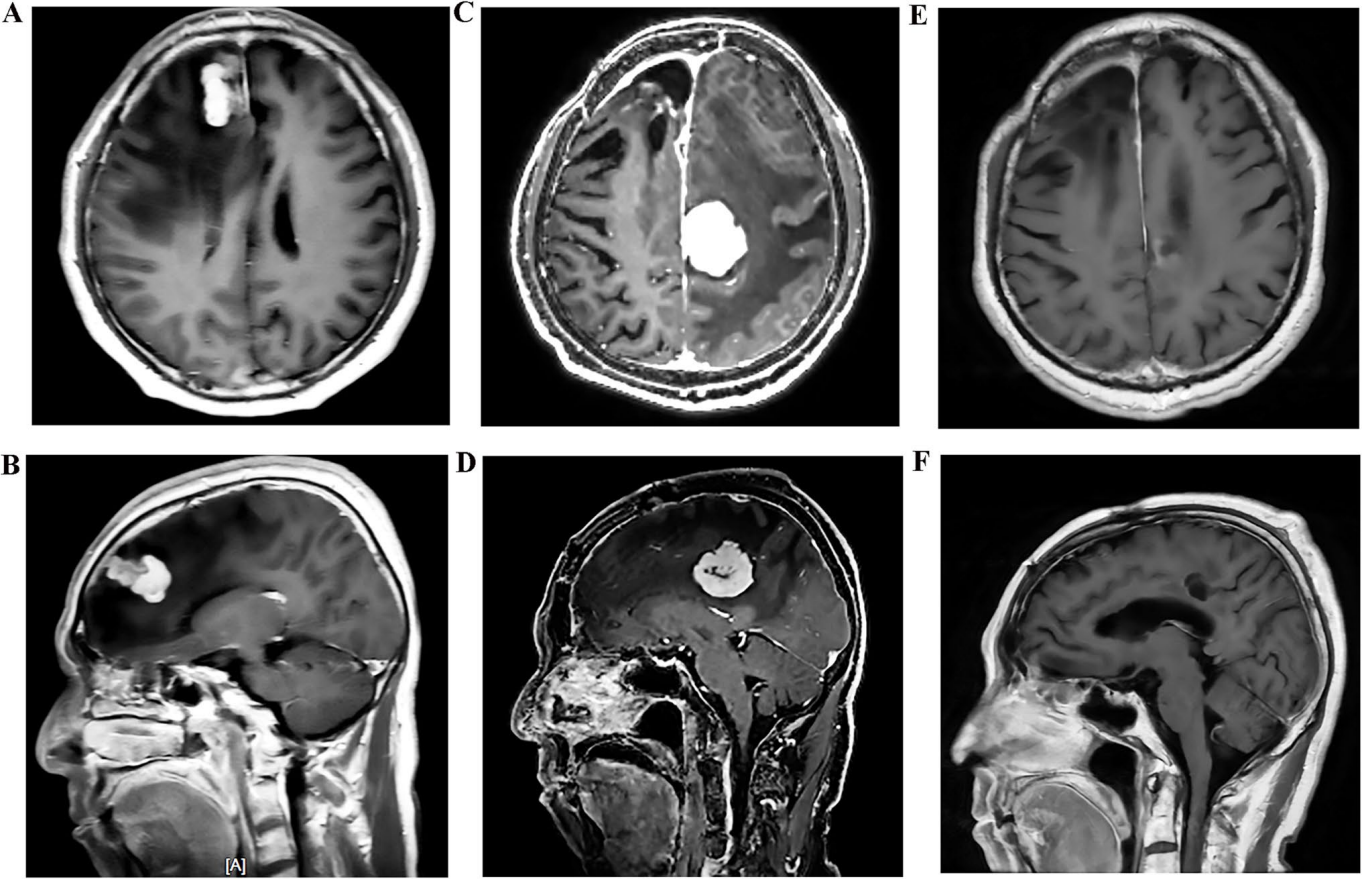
Brain MRI. T1-weighted MRI with gadolinium (A, B) showing an enhancing lesion in the near midline of right frontal lobe with perilesional edema. The pathology of this lesion suggested inflammation lesion. T1-weighted MRI with gadolinium (C, D) demonstrating the new enhancing lesion in the left frontal parietal lobe and was diagnosed as PCNSL. Follow-up T1-weighted MRI with gadolinium (E, F) revealing nearly complete resolution of the new lesion after chemotherapy therapy
